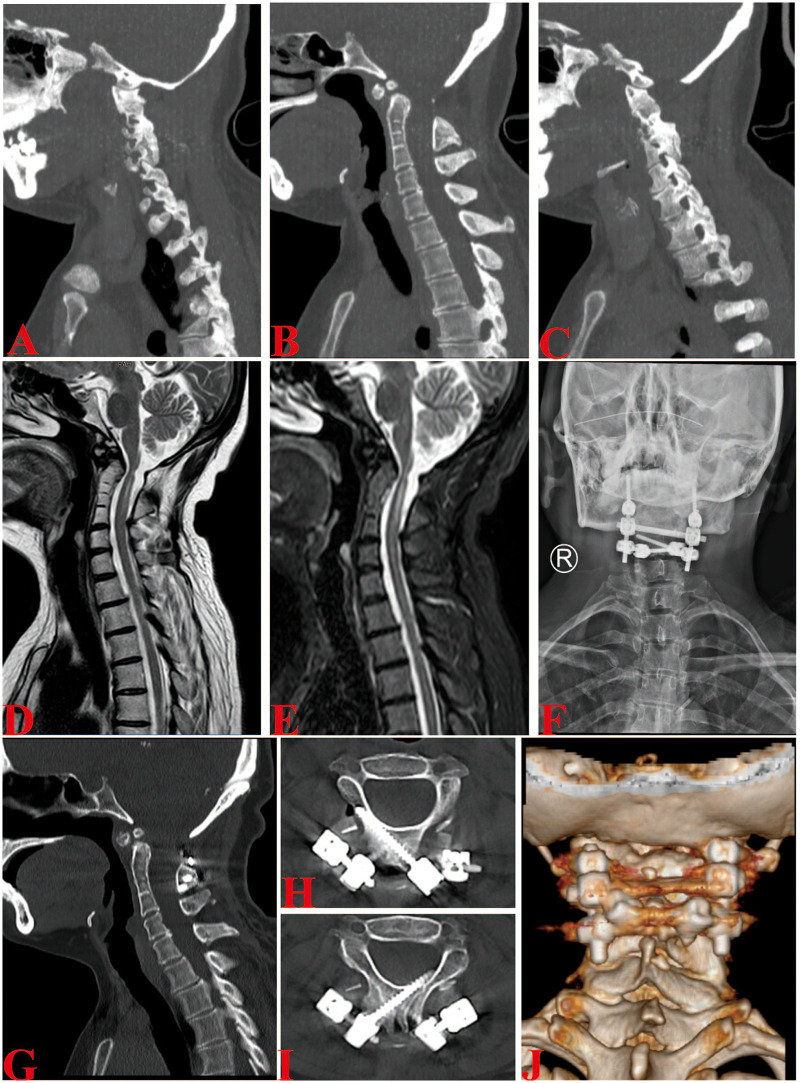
A 45-year-old female: (A–C) preoperative CT showed odontoid process dissociation and atlantoaxial dislocation; (D,E) the spinal cord was obviously compressed and thinned on MRI before operation; (F) x-ray at 3 days after operation: (G–J) it was 2 years after operation, and CT showed no vertebral lamina screws or loosening between screws.
